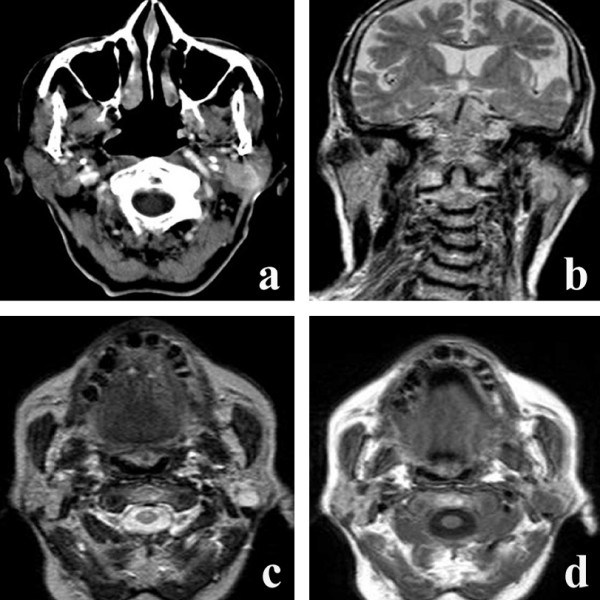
a) Axial contrast-enhanced CT scan shows a well-defined, oval, smooth mass in the deep portion of the left parotid gland, confined in the glandular parenchyma and enhancing. The parotid gland displays a normal morfo-volumetric aspect; b) Coronal T2-weighted MR image. The mass has a homogeneous moderately high signal intensity; c) Axial T2-weighted MR image. The lesion shows well-defined nodular mass with a moderately high signal intensity; d) Axial T1-weighted image of the lesion. It shows a uniform low signal intensity.
